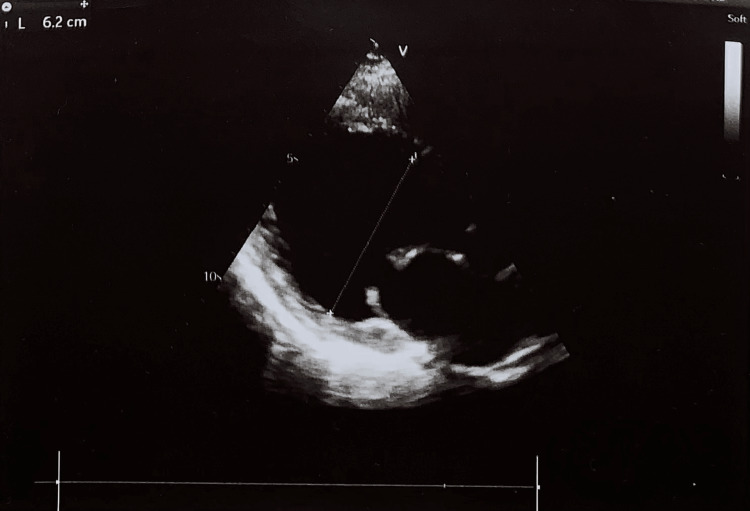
Transthoracic 2D ECHO, parasternal long-axis view showing left ventricle internal dimension in diastole of 62 mm
